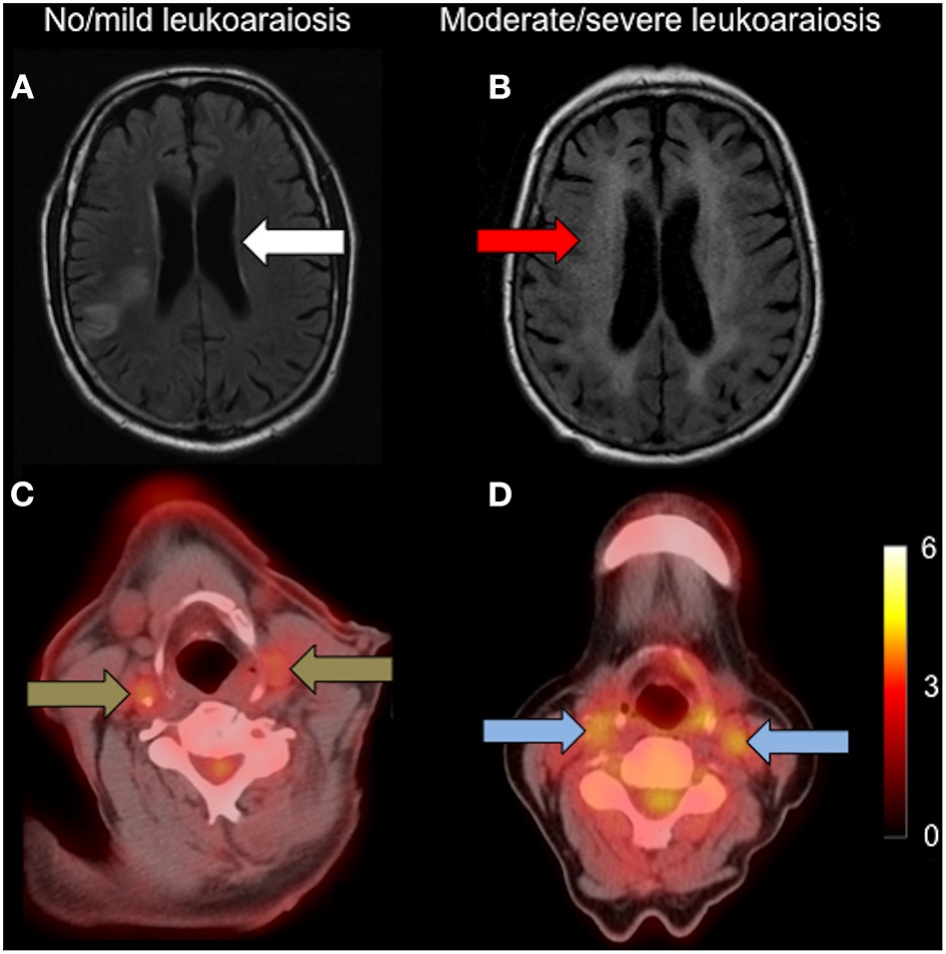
Carotid FDG uptake according to leukoaraiosis severity. Left: (A): axial FLAIR showing no/mild leukoaraiosis (white arrow) with (C): associated low carotid FDG uptake (brown arrows); Right: (B): axial FLAIR showing moderate/severe leukoaraiosis (red arrow) with (D): higher associated carotid FDG uptake (blue arrows). Both FDG-PET/CT images are set to the same scale, with the scale bar showing FDG SUV.
